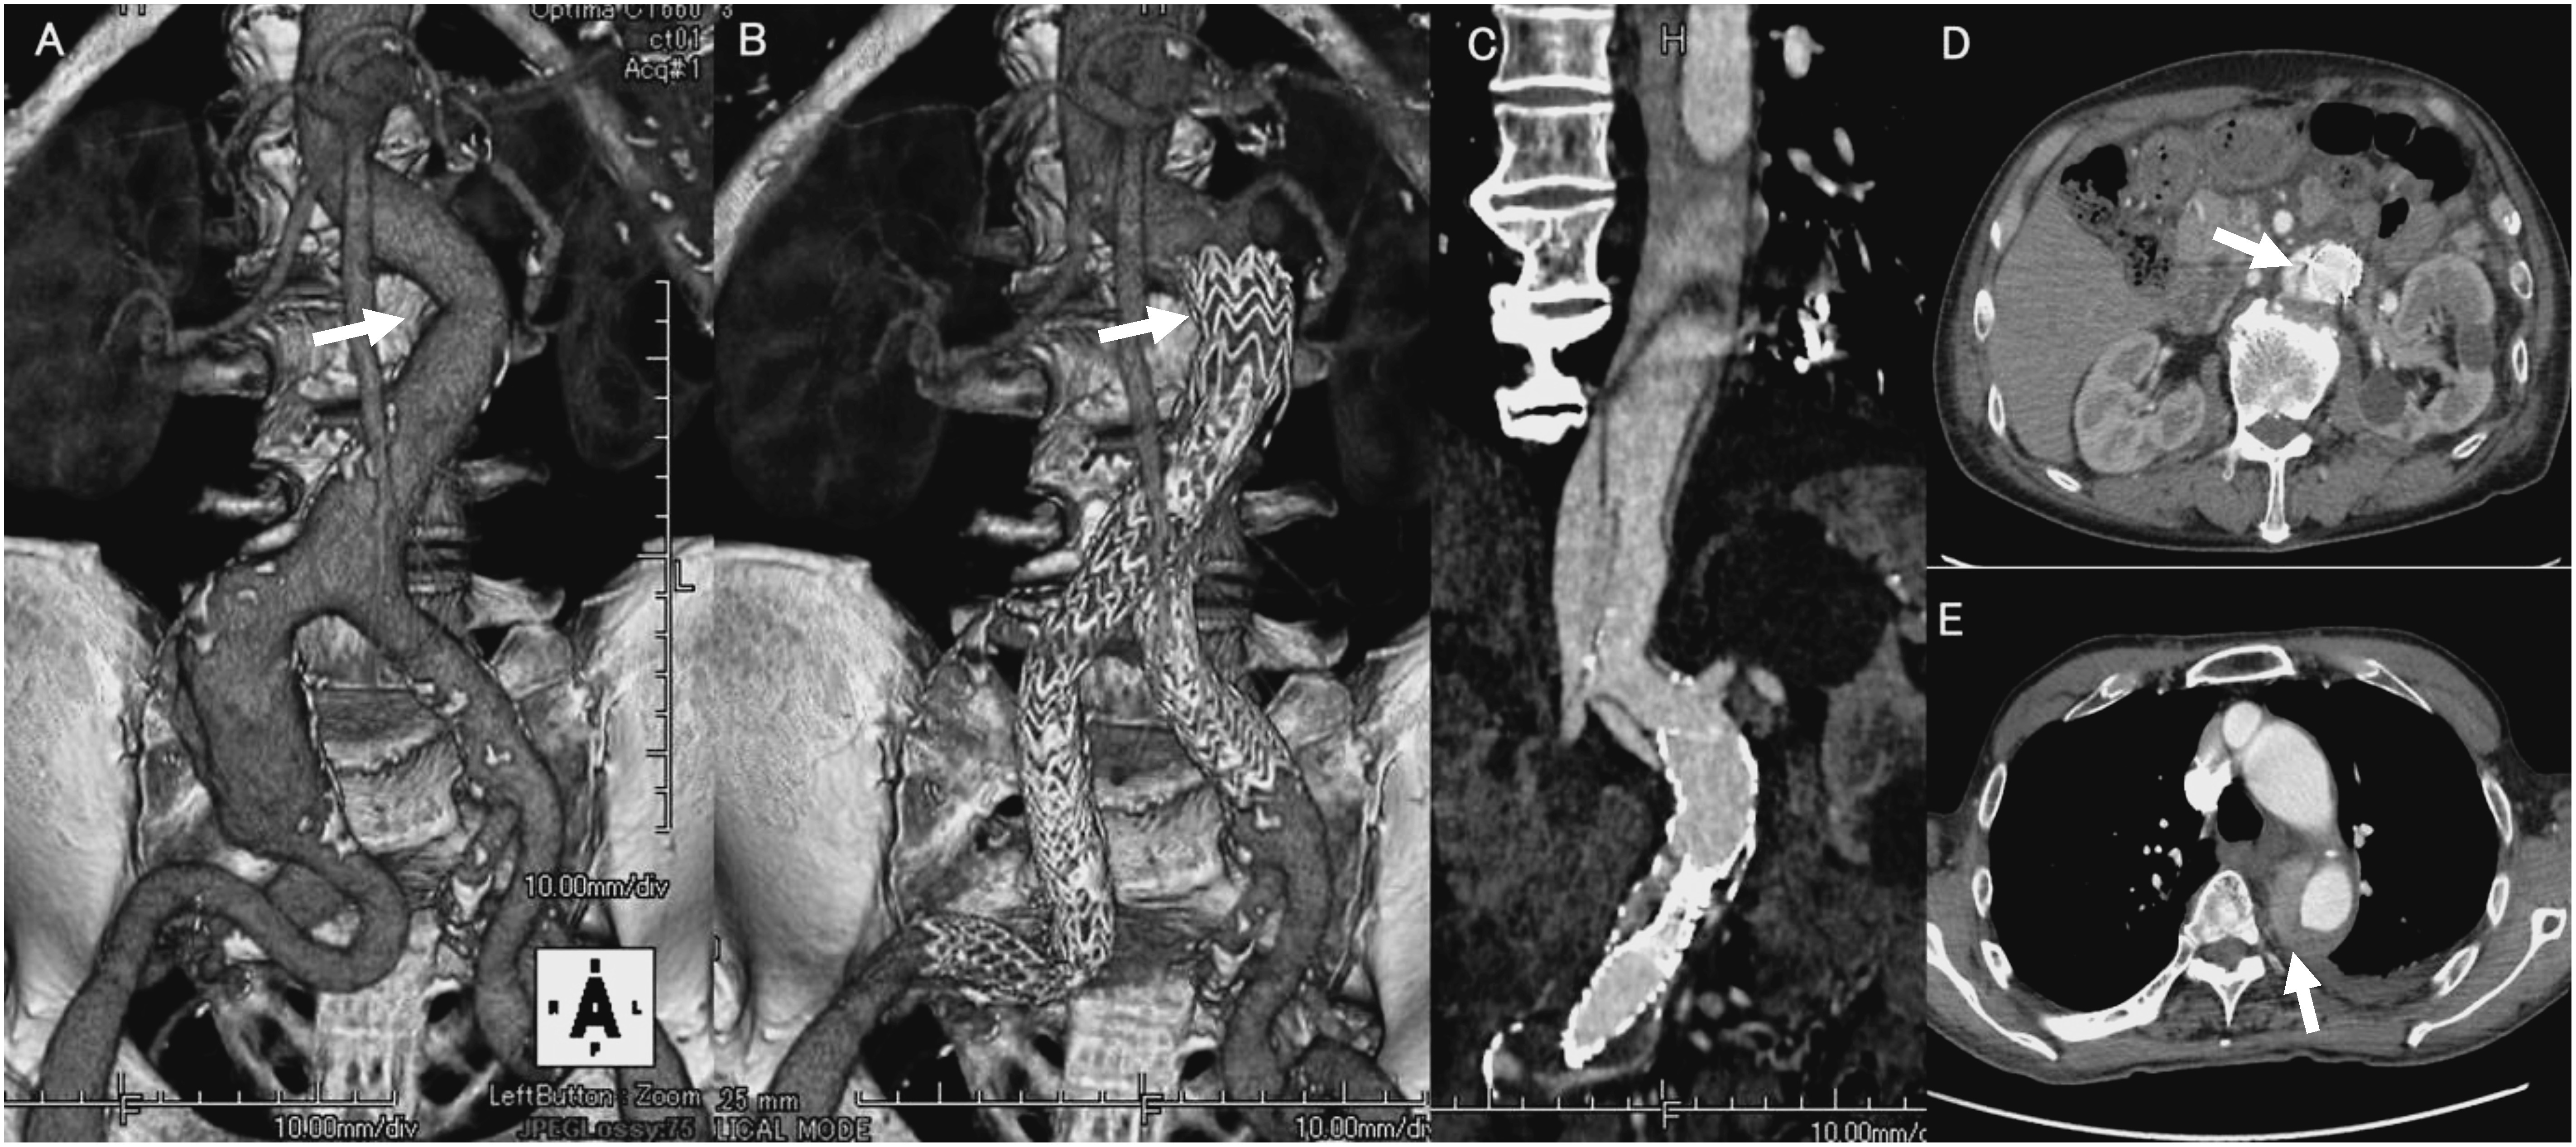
Fig. 1 (A) Preoperative three-dimensional computed tomography angiogram (3DCTA) revealing no calcification or atherosclerosis in the proximal neck. It was found that the infrarenal aortic neck angle was 84° (white arrow). (B–E) Postoperative CTA. (B) 3DCTA showing that the proximal edge of the stent graft was located at the angulated neck (white arrow). (C) Multiplanar reconstruction revealing type B aortic dissection. (D) The axial plane at the proximal edge level of the stent graft. The white arrow shows the dissection entry. (E) The axial plane at the level of the left subclavian artery. The white arrow shows that the false lumen was patent but the contrast medium was diluted.
